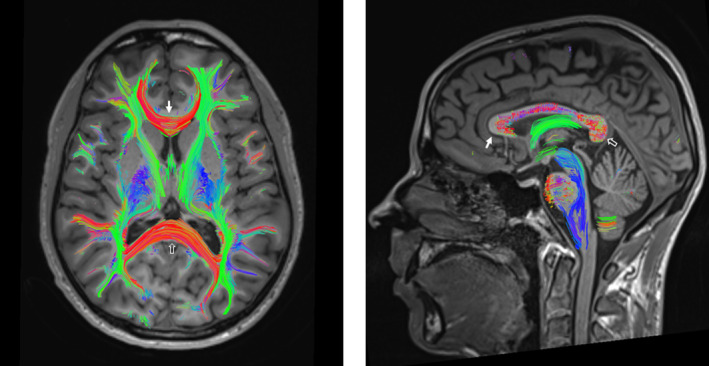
Brain DTI analysis. Tractography overlaid on a 3D GE T1‐weighted MRI of a normal subject, showing fibers in the brain. Due to their right–left orientation, fibers crossing the corpus callosum appear in red. The genu and splenium of the corpus callosum are indicated with solid white and hollow arrows, respectively.
